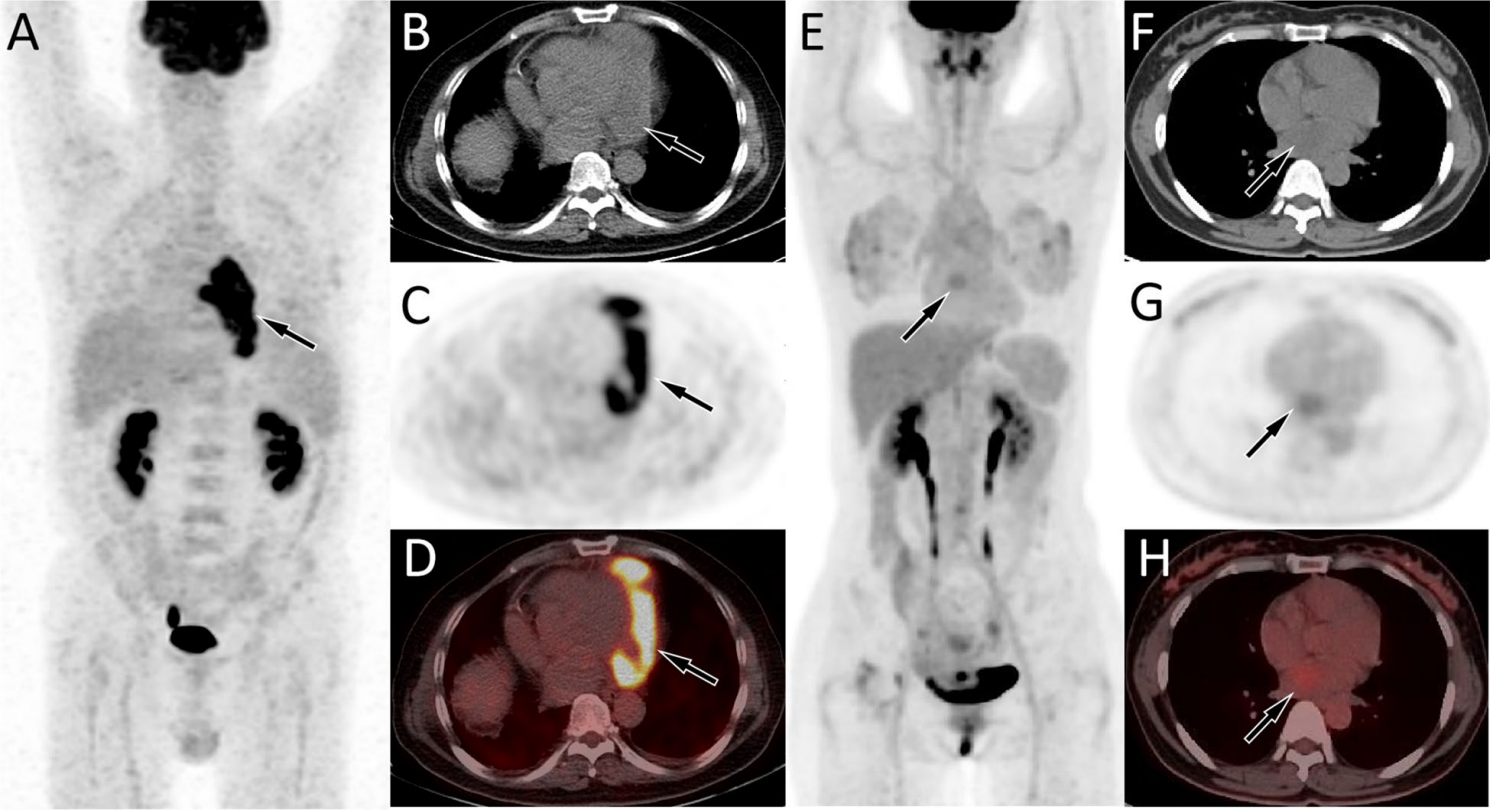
(A–D) A 50 year old male patient underwent CT examination due to breathing difficulties and found an isodensity soft tissue mass in the pericardium. To further evaluate the nature of the mass, PET/CT examination was performed, which was later confirmed by pathology as tuberculous pericarditis. The maximum intensity projection (MIP, A) showed a signifcantly increased ^18^F-FDG uptake in the heart region (arrow). Axial CT (B), PET (C) and PET/CT(D) showed that the lesion with high ^18^F-FDG uptake on the MIP map, which is isodense soft tissue mass located in the left part of the pericardium, with SUVmax, SUVmean, and MTV being 11.06, 7.12, and 25.43, respectively (arrows). (E–H) A 47-year-old woman with palpitation and dyspnea underwent echocardiography to find a right atrial mass. PET/CT was performed to further evaluate the nature of the tumor, which was pathologically confirmed as myxoma. The MIP map showed a lesion with slightly increased ^18^F-FDG uptake in the heart region (E, arrow). Axial CT (F), PET (G) and PET/CT (H) showed that the lesion was isodensity and located in the right atrium, with SUVmax, SUVmean, and MTV being 3.2, 1.7, and 6.48, respectively (arrows).
